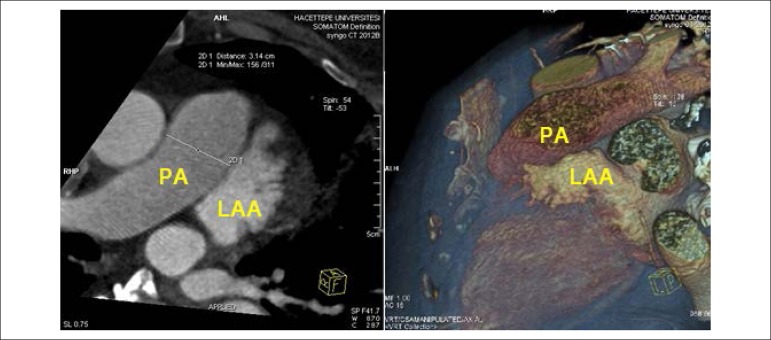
Computed tomography image of the neighborhood between pulmonary artery and left atrial appendage.
